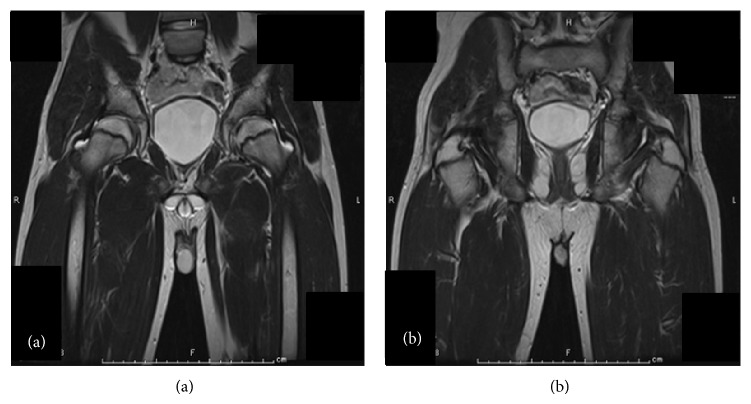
Coronal T2 weighted image in MRI shows no evidence of avascular necrosis of the femoral head (a) and healing of soft tissue damage (b) at 6 weeks.
